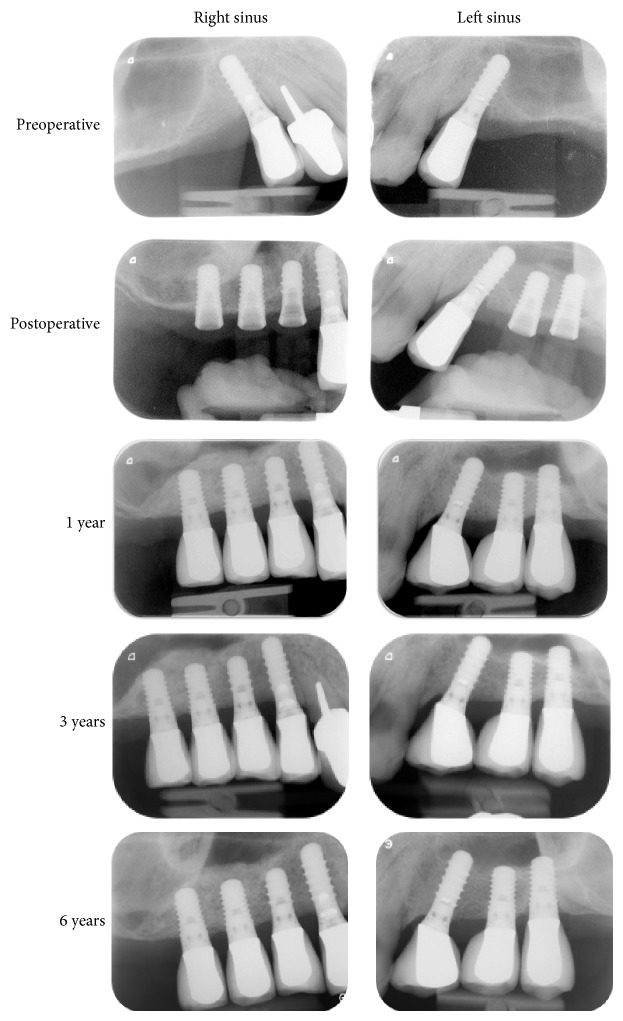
Periapical radiographs. The 8 mm tapered implants were placed using the osteotome sinus floor elevation procedure with grafting material (right sinus) and without grafting material (left sinus). In all controls after surgery, implants were clinically stable. A radioopaque area corresponding to the augmented sinus floor was present around the implants placed without graft and around and above the implants placed with graft.
